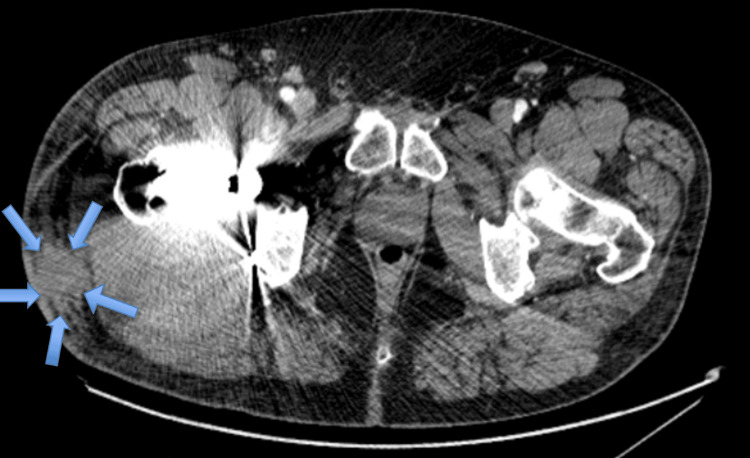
Axial CT showing a right lateral soft tissue hematoma adjacent to the hip arthroplasty. An axial CT image of the pelvis showing a right lateral soft tissue hematoma (blue arrows) adjacent to the total hip arthroplasty components. No pseudoaneurysm is identified.
